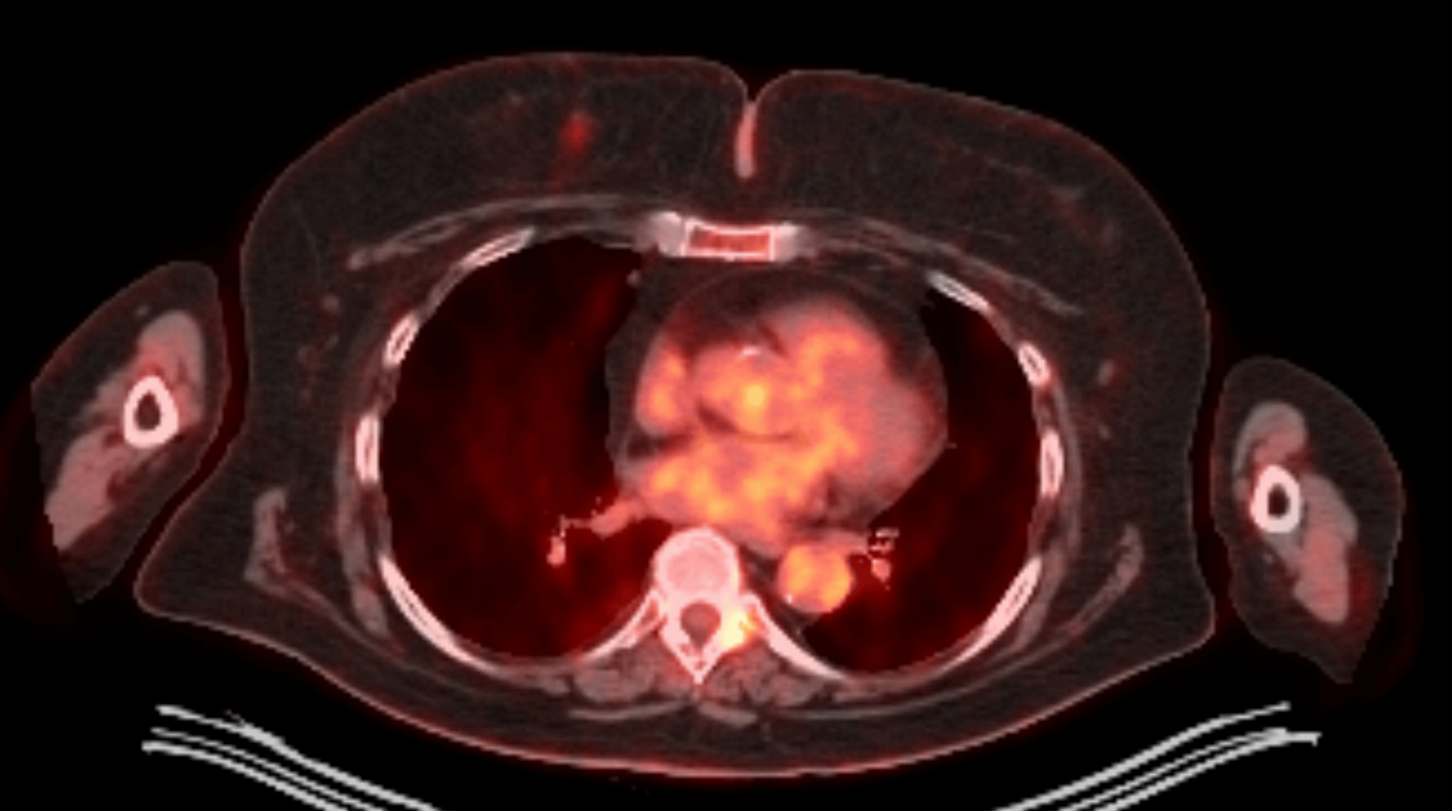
PET-CT showing axial view of the breast, axillary, and thoracic area. PET demonstrates an avid lesion at the one o'clock position in the right breast. There are no PET-avid lymph nodes in the axilla. This one o'clock lesion in the breast was biopsy-proven to be marginal zone lymphoma.
